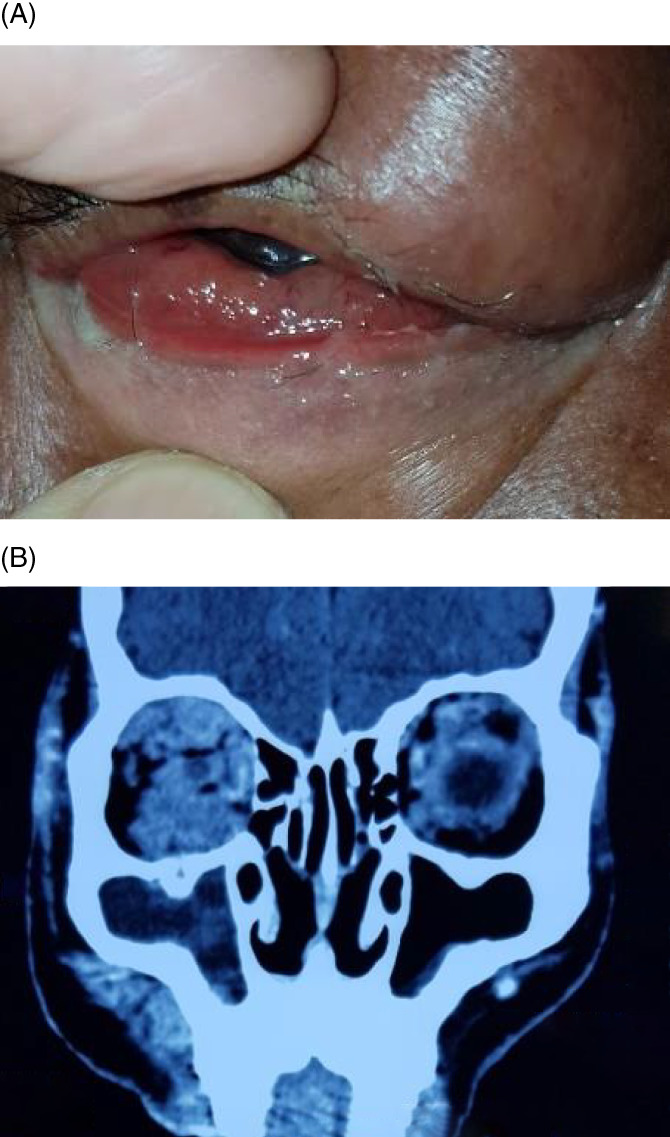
Orbital infiltration of Non‐Hodgkin lymphoma. (A) Conjunctival and orbital lymphoma. (B) CT scan image showing bilateral diffuse orbital infiltration
